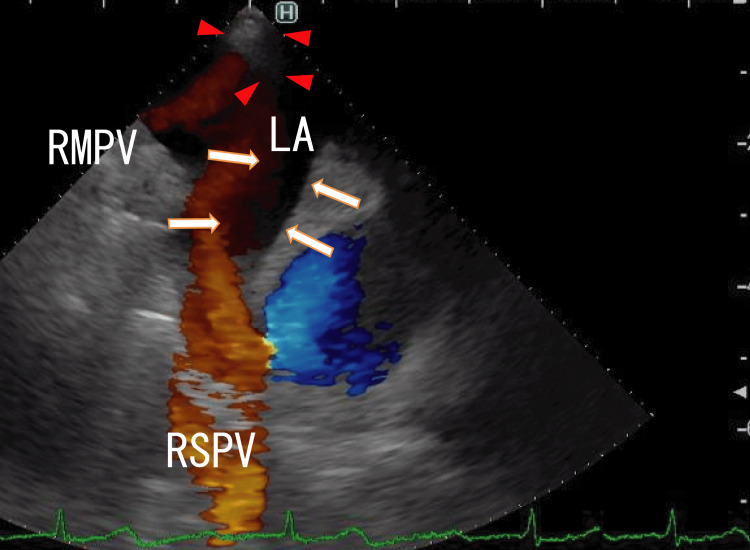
TEE images obtained after five months of standard-dose warfarin remedy showing thrombi in the LA TEE images obtained after five months of standard-dose warfarin remedy showing thrombi in the LA that appeared to be linked to thrombi in the RSPV (arrows). The thrombi included no white areas. Thrombi expanded from the RIPV and were depicted as dim white areas that looked like to be decreased (arrowheads). LA, left atrium; RMPV, right middle pulmonary vein; RSPV, right superior pulmonary vein; TEE, transesophageal echocardiography
